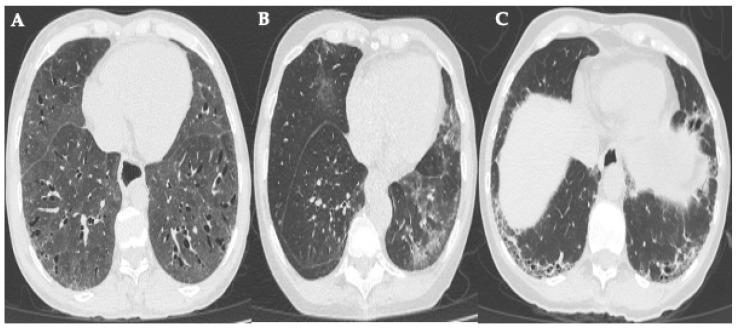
High-resolution axial computed tomography images with different patterns of ILD (Source: Radiology department of Ramon y Cajal’s Hospital). The images show patterns of non-specific fibrotic interstitial pneumonia (A,B), highlighting the presence of ground-glass opacities and traction bronchiectasis. Image (C) shows usual interstitial pneumonia, where the previous findings and a pattern of honeycombing can be seen. The dilated esophagus suggests ILD related to connective tissue disease rather than idiopathic pulmonary fibrosis.
